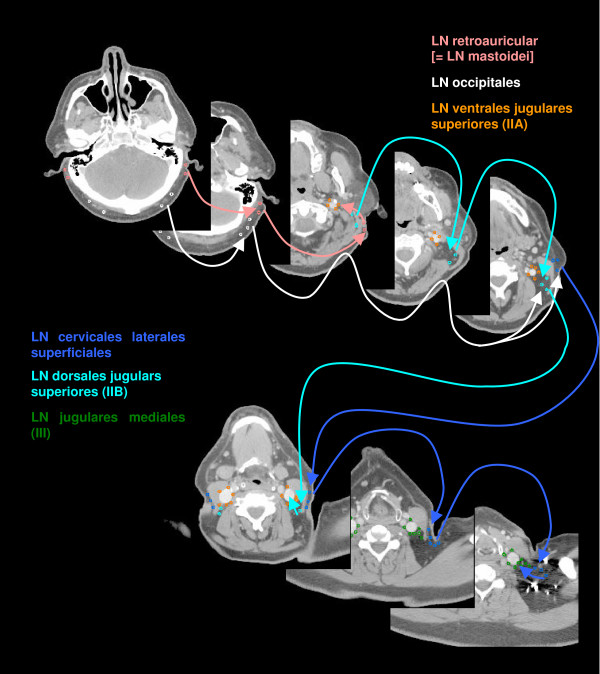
Lymph regions and drain contoured in transversal CT slices: LN occipitales (white), LN retroauriculares [ = LN mastoidei] (pink), LN cervicales laterales superficiales (medium blue) and LN dorsales jugulares superiores (cyan) [1.8 cm slice thickness].
